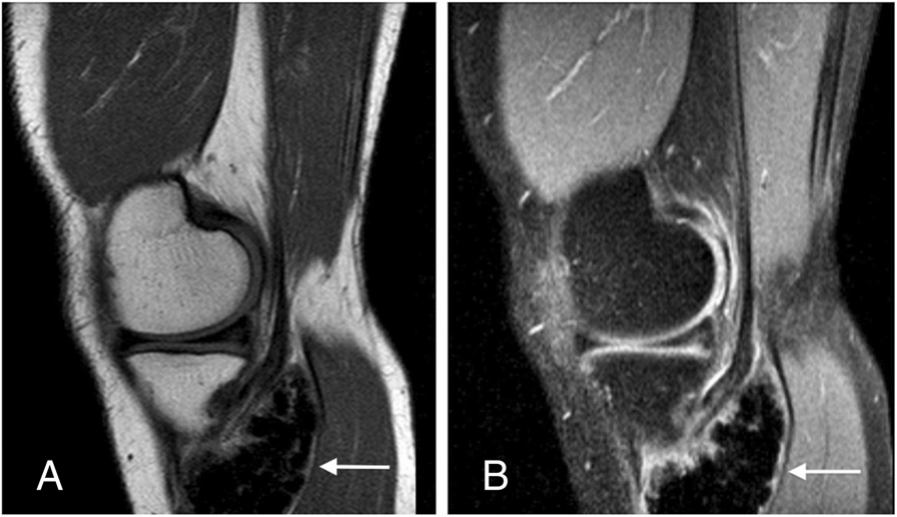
T1-weighted (A) and T2-weighted (B) sagittal MR image of the lesion (arrow).
